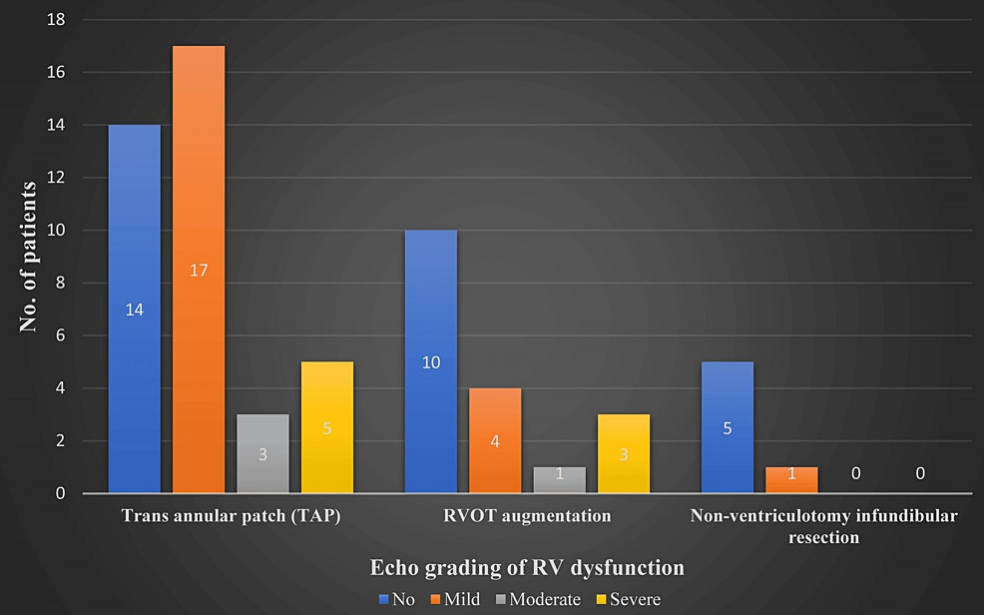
Grades of right ventricular dysfunction in postoperative echocardiography. TAP = transannular patch; RVOT augmentation = right ventricular outflow tract pericardial patch augmentation; RV = right ventricular
